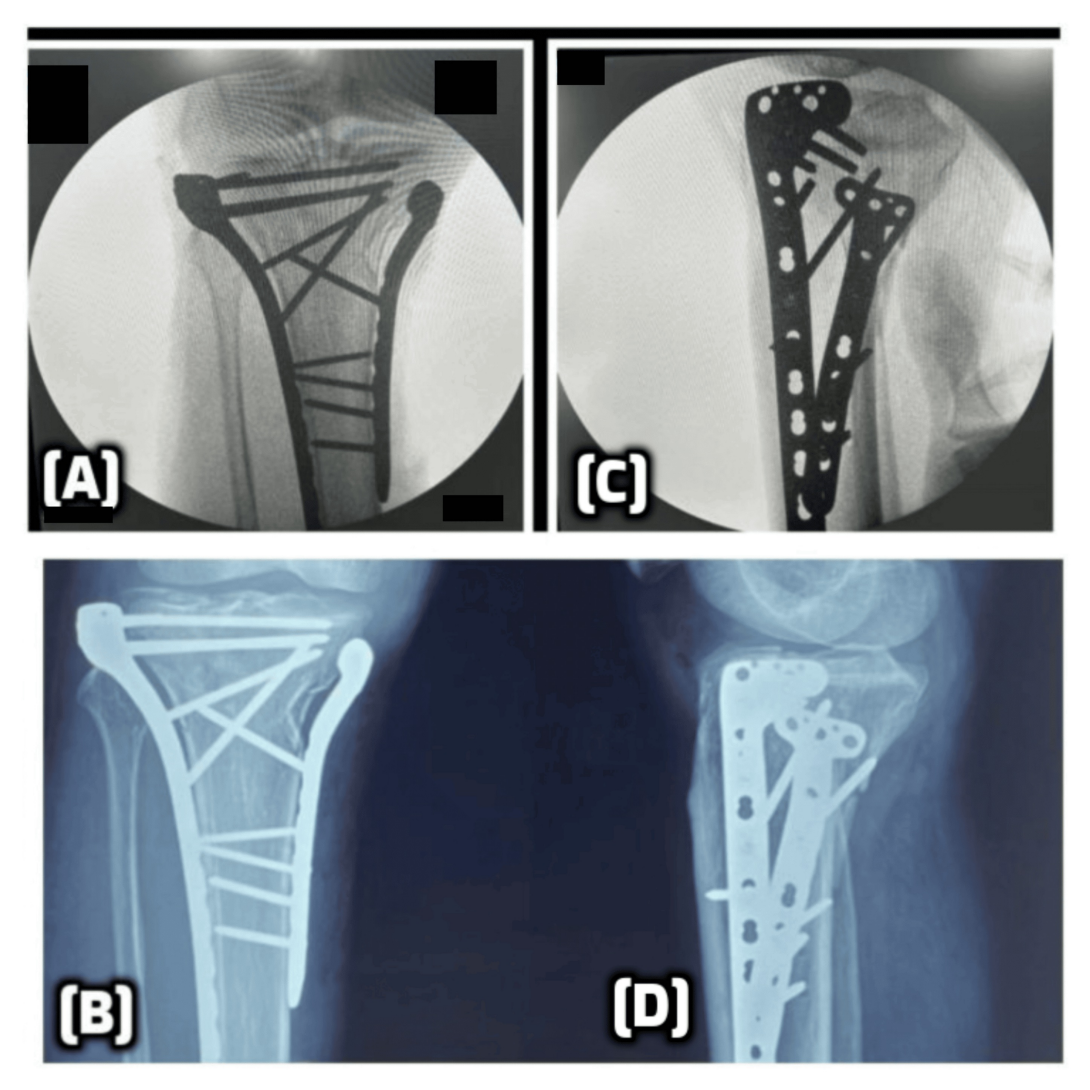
Intraoperative and postoperative radiographic views following dual plating of a bicondylar tibial plateau fracture (A, C) Intraoperative fluoroscopic images showing fixation of a bicondylar tibial plateau fracture using a dual plating technique via a two-incision approach. (B, D) Immediate postoperative radiographs in AP and lateral views demonstrate anatomical reduction and stable fixation with lateral and medial LCP. LCP: locking compression plates; AP: anteroposterior
